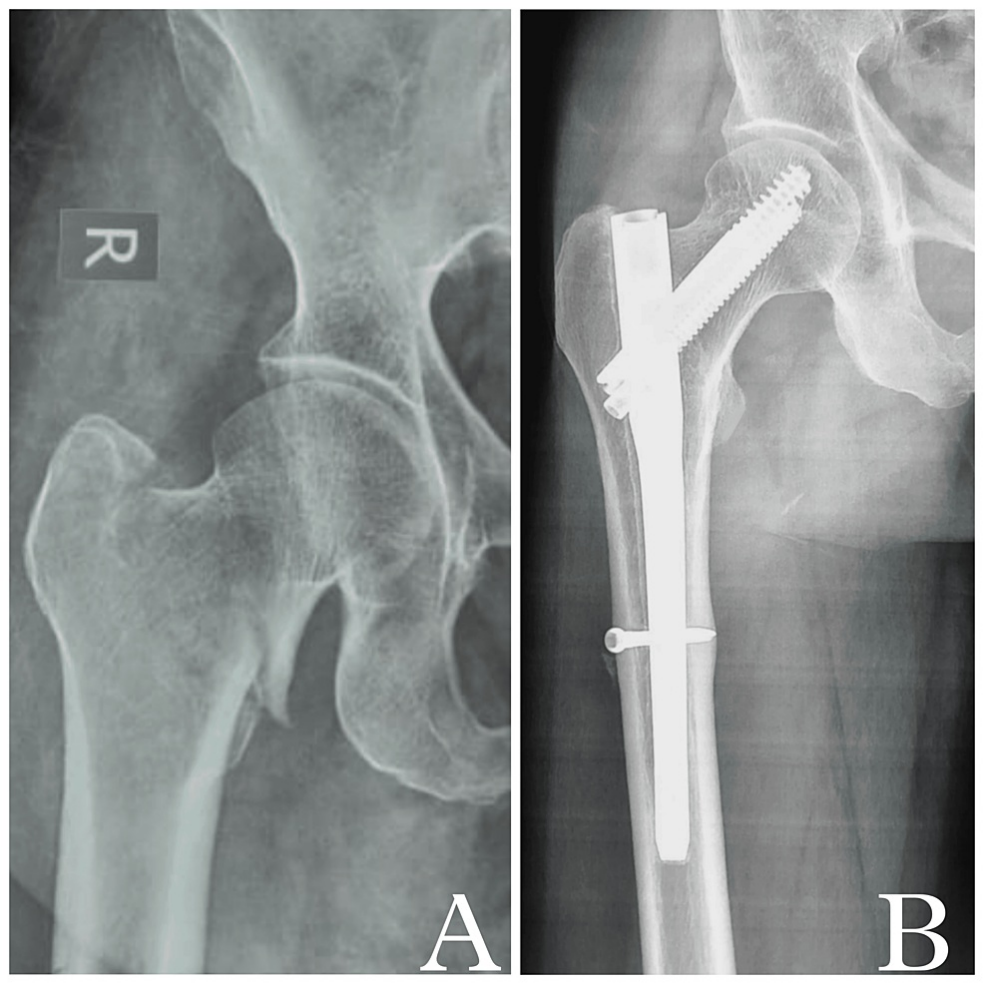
Intertrochanteric fracture fixation using short InterTAN nail (A) Preoperative radiograph showing right intertrochanteric fracture. (B) Two-year follow-up X-ray showing fracture union. Note there is no implant loosening, screw back-out, or collapse of fracture.
